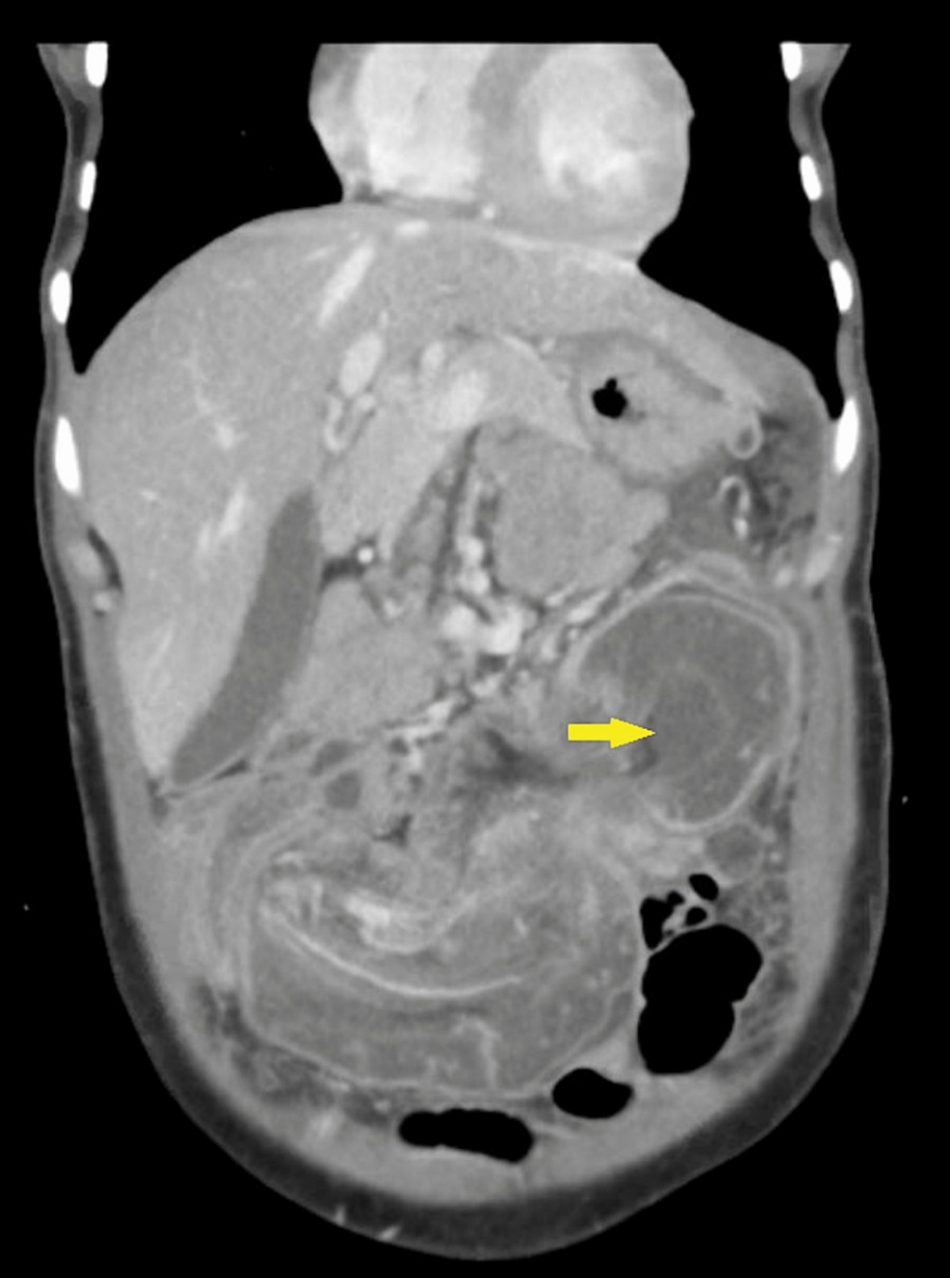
Transverse colo-colonic intussusception in the coronal cut of abdominal computed tomography scan showing “sausage sign” (yellow arrow)
